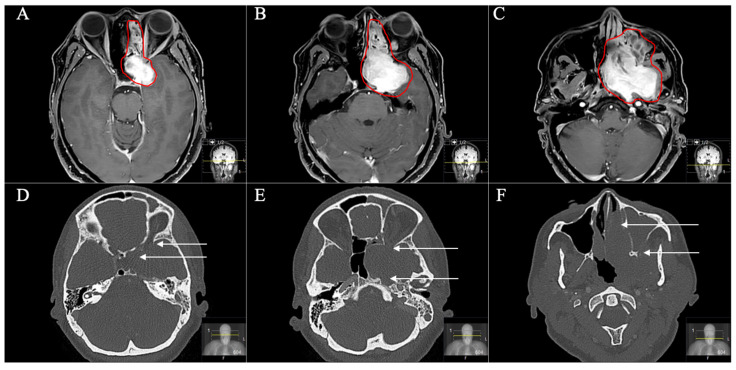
A 54-year-old female patient presented with slowly progressive visual impairment on the left side, trigeminal hypesthesia (V3) and an abducens nerve palsy with intermittent facial pain. (A–C) Preoperative axial T1-weighted gadolinium-enhanced MRI showing a massive space-occupying mainly extracranial trigeminal schwannoma (Type D) involving the middle fossa up to the orbital funnel. The schwannoma is bounded medially by the lamina papyracea, inferiorly by the hard palate and infiltrating the pterygopalatine fossa laterally. The middle nasal concha forms the anterior border. The paranasal sinuses are completely misplaced. (D–F) Preoperative axial CT scans demonstrating the destruction of the anterior skull base including the orbital funnel, the foramina ovale and rotundum and also the nasal septum and the maxillary sinus (arrows).
